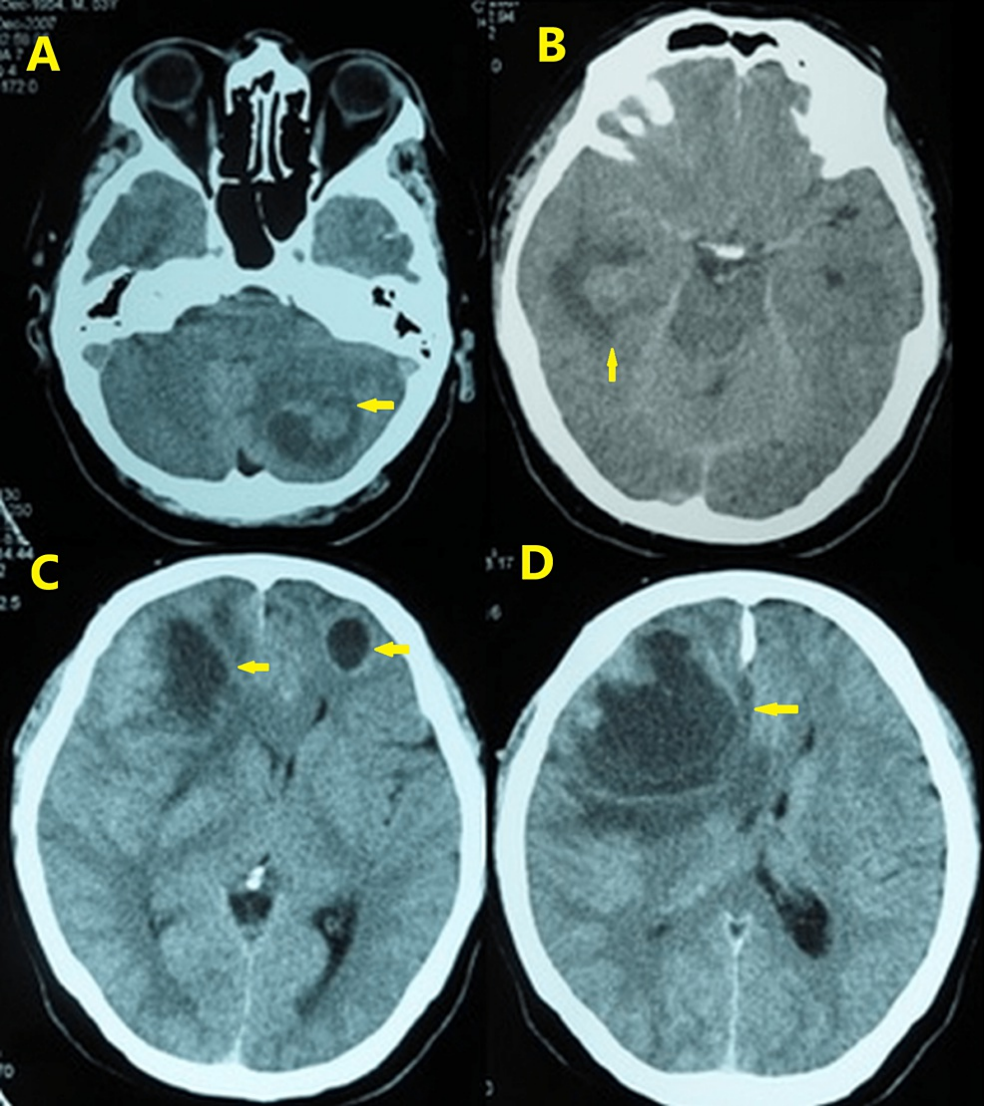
Brain computed tomography on December 31, 2007. Post-contrast medium axial images displaying intracranial metastases as low-density mass-like lesions with heterogeneous or ring-like enhancement and perifocal edema in the left cerebellar hemisphere (A), right temporal lobe (B), and bilateral frontal lobes (C, D). The size of the metastatic lesion in the right frontal lobe (D) is 5.5 cm × 6.2 cm with perifocal vasogenic edema, mass-effect toward the frontal horn of the right lateral ventricle, and midline shift to the left.
